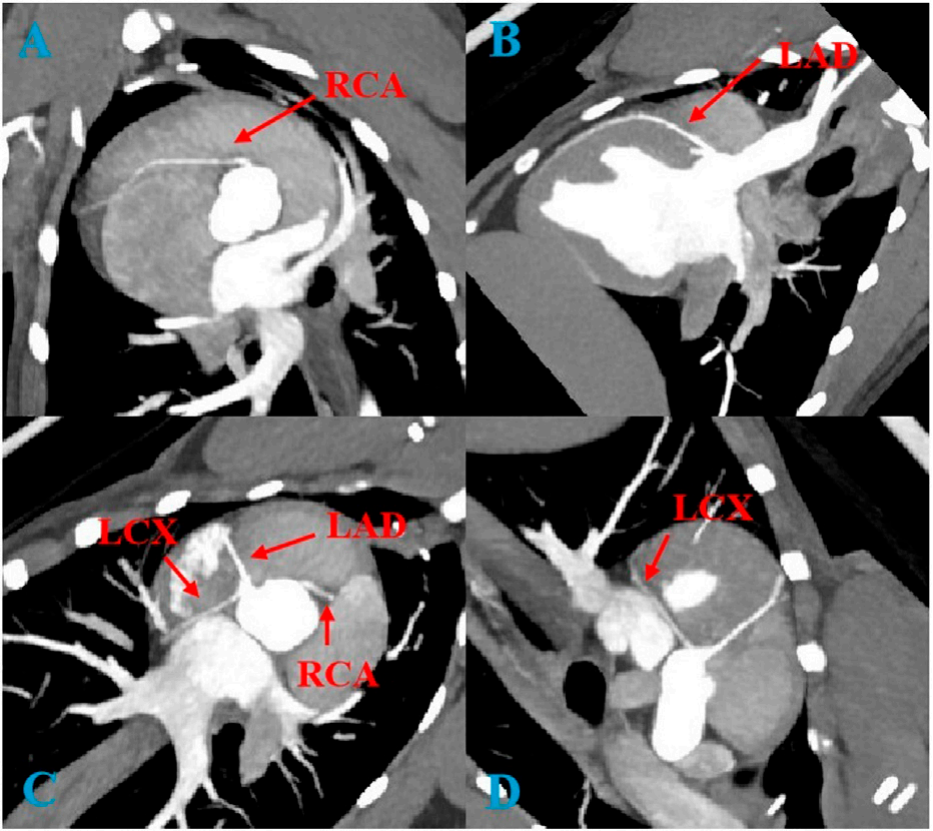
Coronary CTA images of a Beagle receiving 6 ml of contrast agent. (A): RCA, right coronary artery; (B): LAD, left anterior descending artery; (C): Three main coronary arteries; (D): LCX, left circumflex artery.
